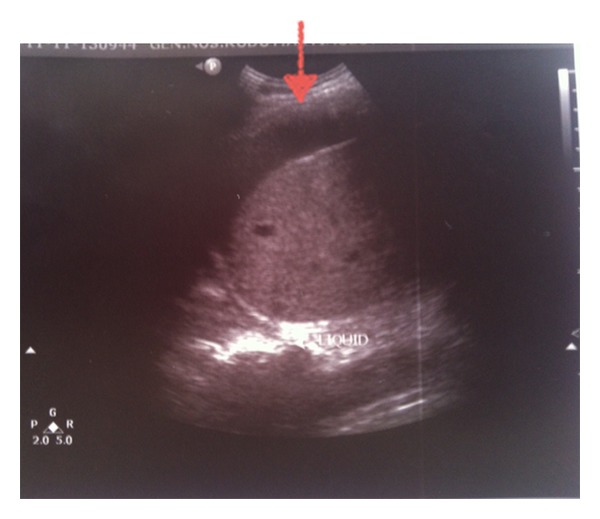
Ultrasound examination of the abdomen showing a hypoechoic subcapsular collection of the liver (arrow).
